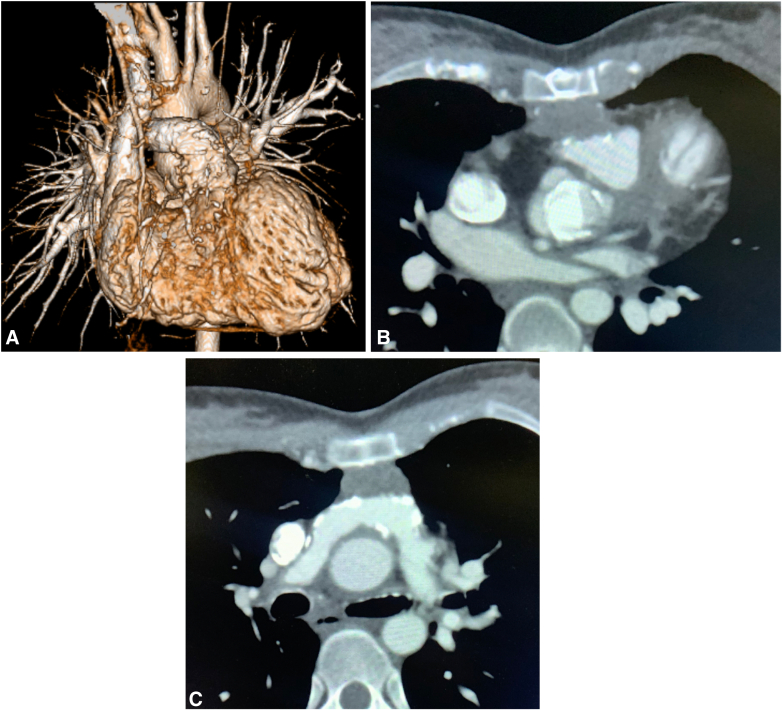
Three-dimensional computed tomography imaging (A), and axial view demonstrating compression of the right ventricular outflow tract by a depressed sternum (B) and severe calcification of the bilateral pulmonary arteries (C).
